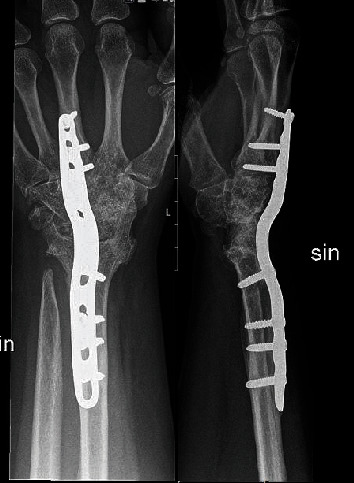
Radiographs 3 years postoperatively with bony union.
